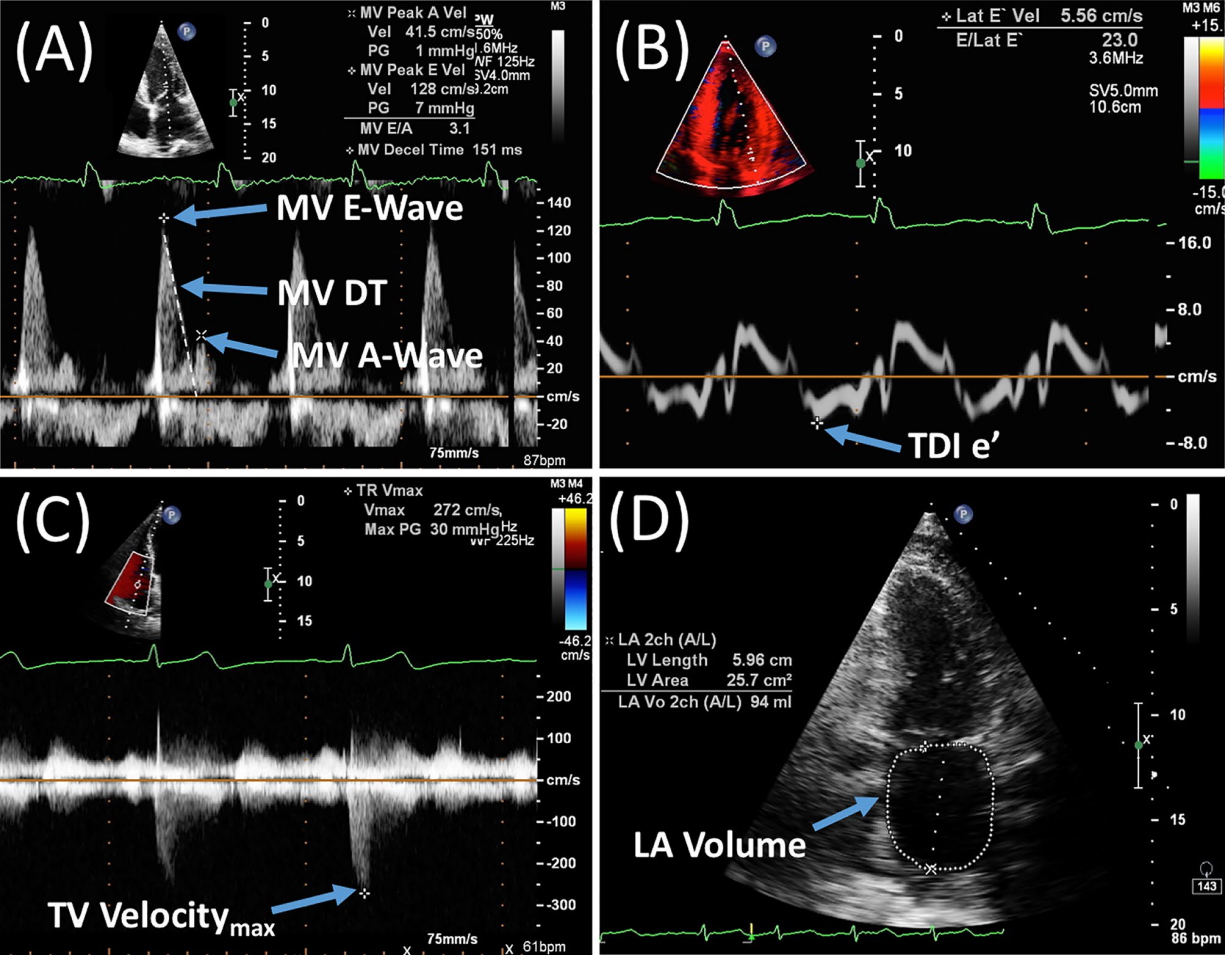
Echocardiographic measures used to assess diastolic dysfunction. a Mitral valve (MV) E-wave and A-wave peak velocities, obtained on pulse-wave Doppler, are combined to generate the E/A ratio. b Mitral valve E-wave peak velocity and tissue Doppler (TDI) *e*ʹ velocity (here measured at the lateral MV annulus; average *e*ʹ is calculated from both the lateral and medial sites) are combined to generate the *E*/*e*ʹ ratio. c Peak velocity of tricuspid valve (TV) regurgitation. d Apical 2-chamber view and measurement of left atrial (LA) volume. DT–deceleration time
